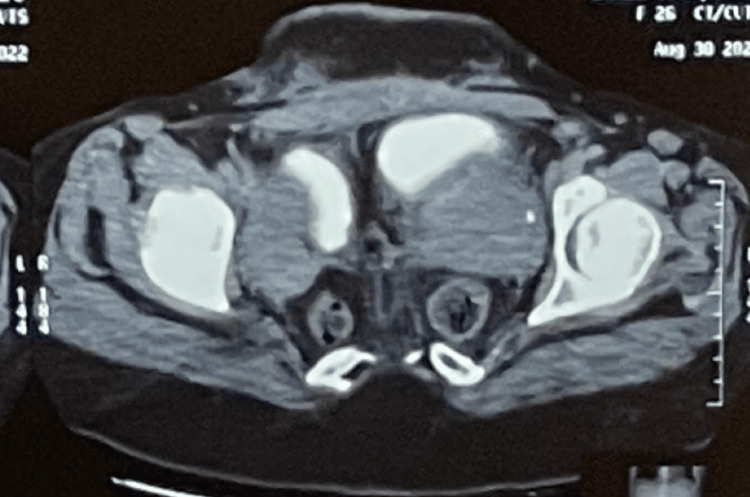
CT scan of pelvis showing duplication of pelvic viscera CT: computed tomography
